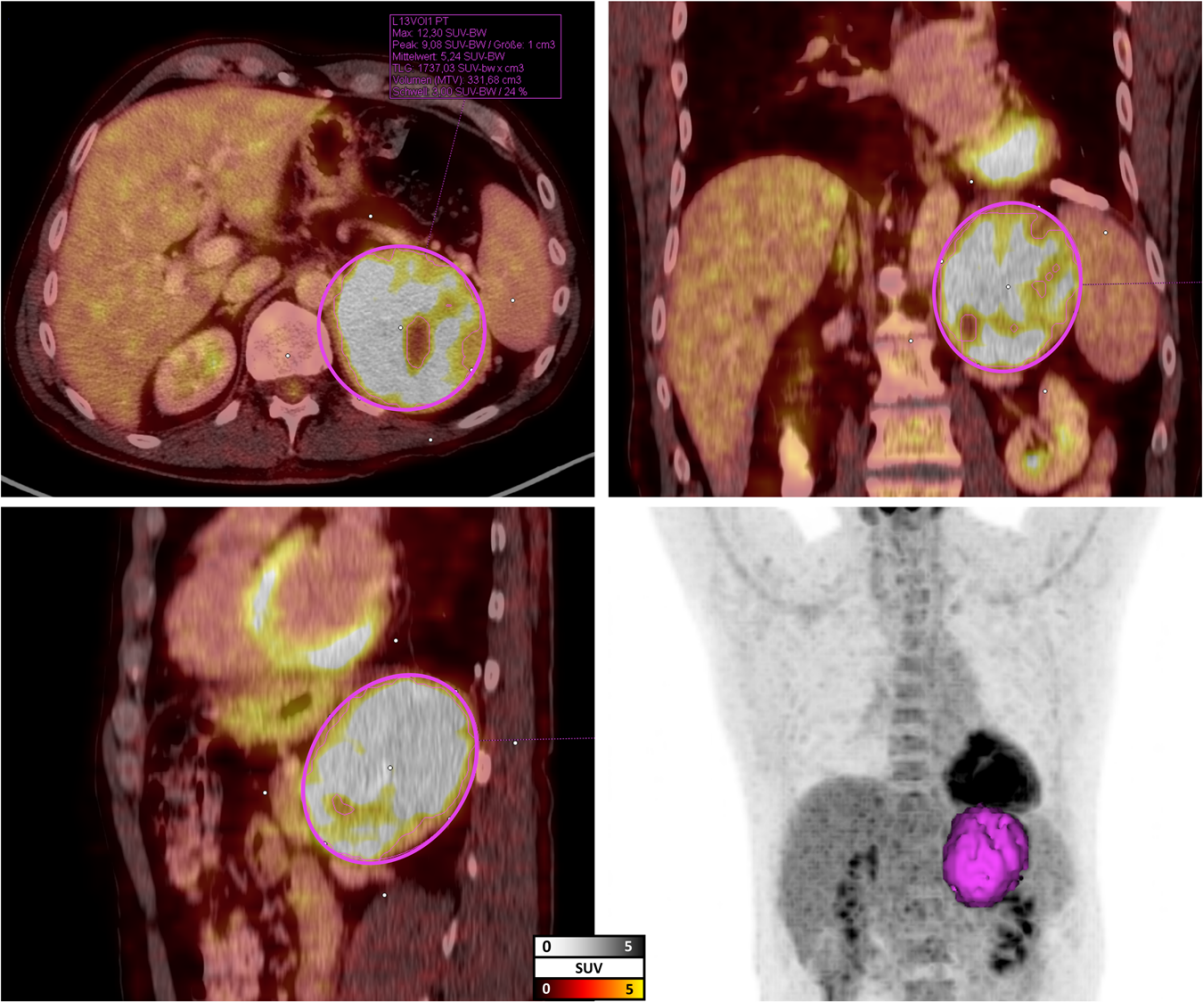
Example of segmentation of the primary tumor. An isocontour volume of interest (VOI) using a threshold SUV of 3.0 was drawn using a three-dimensional segmentation method allowing for a semi-automatic volumetric assessment (measurement results of the patient example: SUV_max_ 12.3. SUV_peak_ 9.08. SUV_mean_ 5.24. TLG 1737.03. MTV 331.68)
